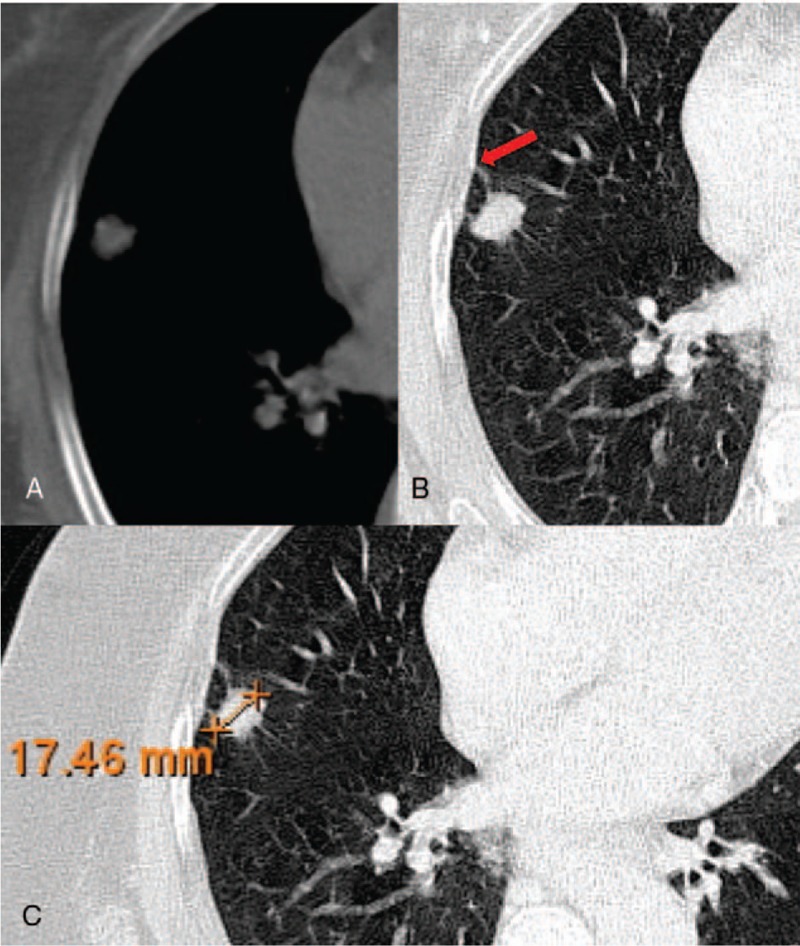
A 69-year-old woman with EGFR-mutated in exon 19 of lung cancer in the right upper lobe, and CT images show a small maximum-diameter mass about 17 mm (C) with obvious pleural retraction (B, red arrow), the absence of emphysema and fibrosis (A). EGFR = epidermal growth factor receptor.
